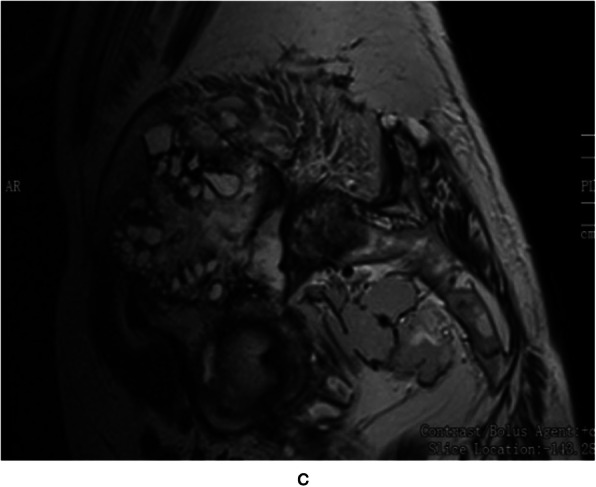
MRI sagittal T2 showed multiple rounded and irregular long T2 signals of different sizes
